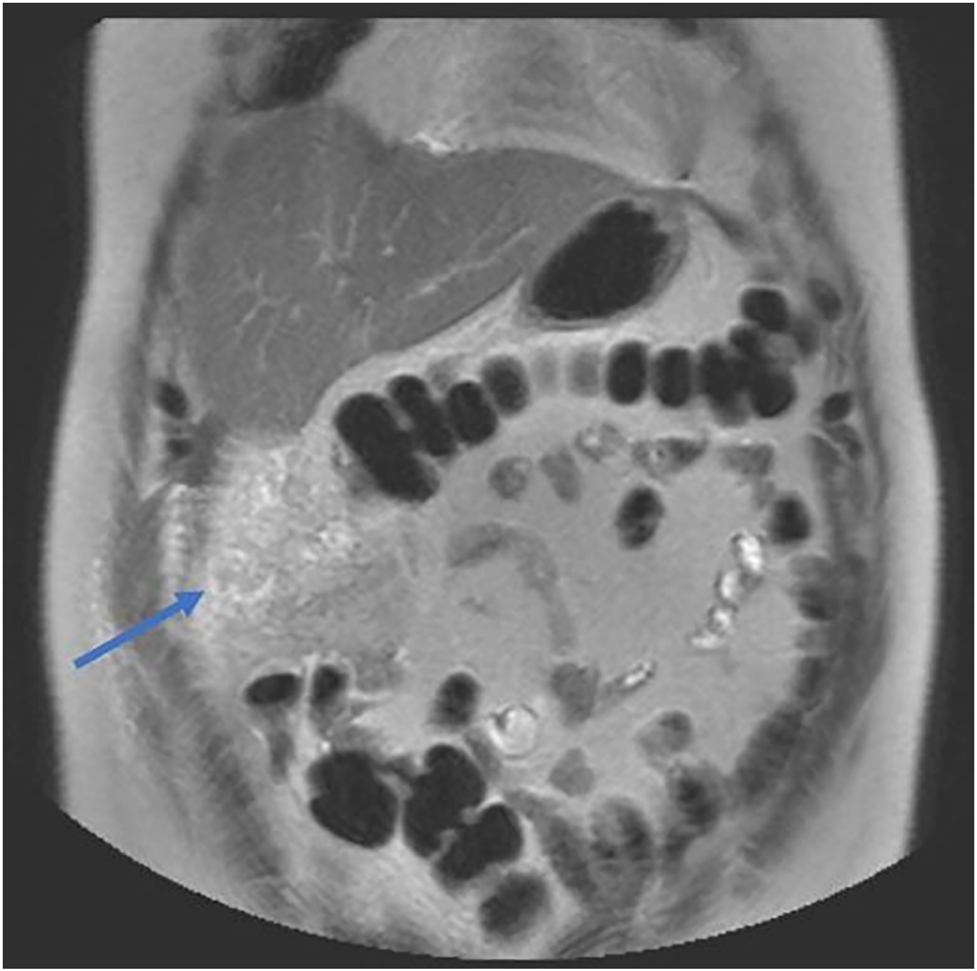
MRI T2 phase showing ill-defined roughly triangular area of altered signal intensity (blue arrow) appearing heterogeneously hyper intense in the omentum of right lumbar region just inferior to the right lobe of liver.
